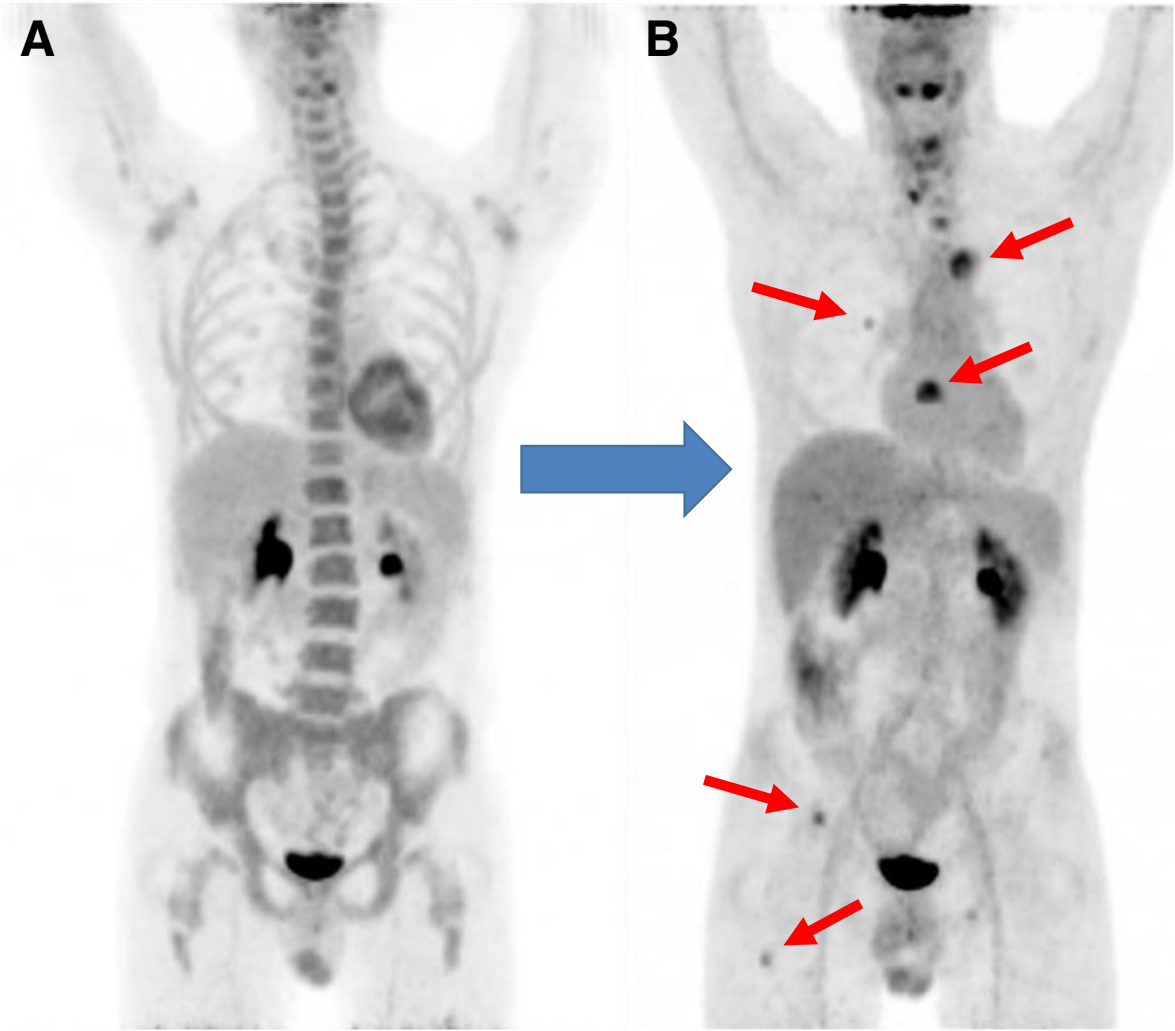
Systemic disease progression evidenced in ^18^F-fluorodeoxyglucose positron emission tomography-computed tomography maximum intensity projection images. Maximum intensity projection fluorodeoxyglucose-positron emission tomography-computed tomography whole-body images from baseline before second-line chemotherapy (a) and 1 month after end of treatment (b). Image a shows no evidence of systemic disease. Image b shows disease progression with new bone and pulmonary fluorodeoxyglucose-avid metastasis (*arrows*)
